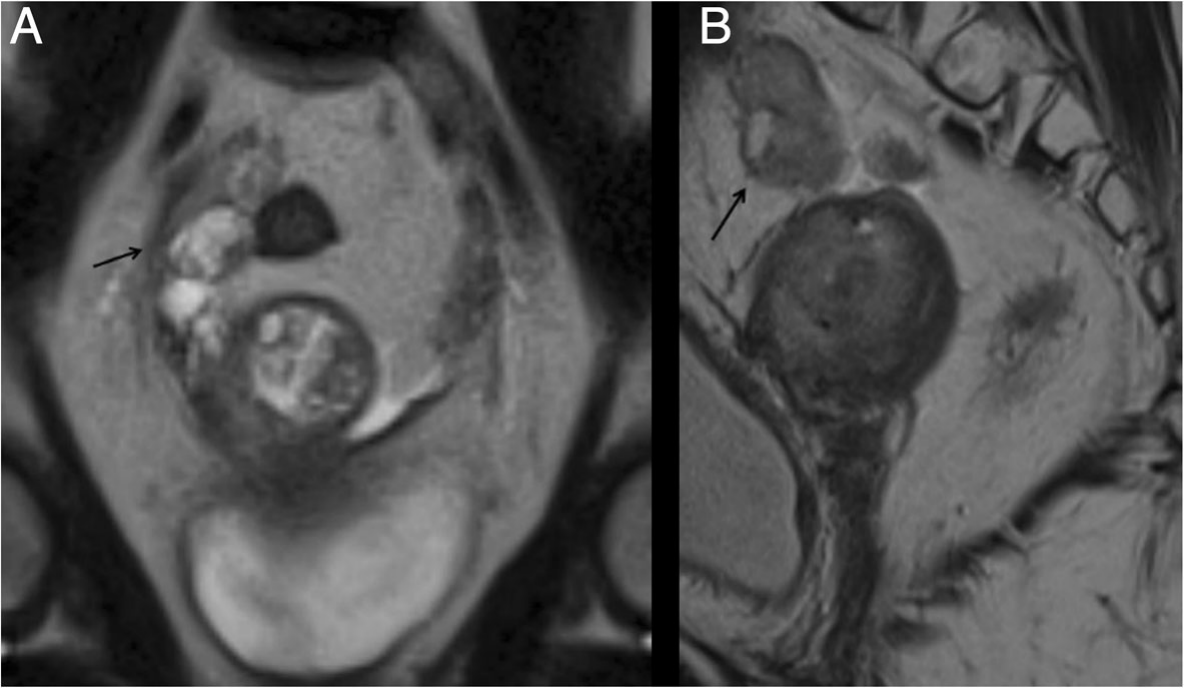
A 54-year-old EC patient with deep myometrial infiltration and serosal involvement. Coronal (a) and sagittal (b) MR images show a small mass in the right ovary (arrows) with solid components of the same signal intensity as the EC, representing ovarian involvement
